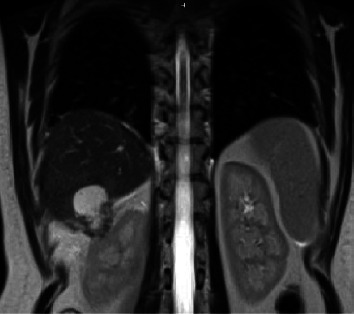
MRI showing RUQ complex cystic mass in the liver extending into the R renal fossa.
